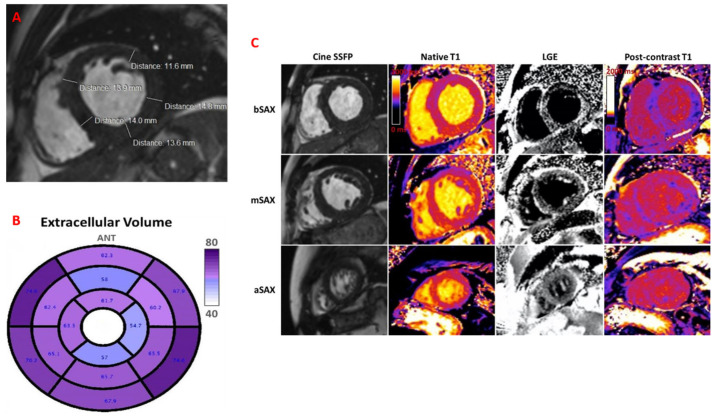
Cardiac magnetic resonance images. (A) Short-axis view showing left ventricular hypertrophy (IVS = 13.9 mm, AWT = 13 mm, LWT = 14.8 mm, PWT = 14 mm). (B) Extracellular volume (ECV) bull’s eye plot evaluated by cardiac magnetic resonance, using a 16-segment model, displaying a progressive decrease of the ECV from base to apex, explaining the substrate of apical sparing deformation pattern. (C) Short axis views at basal (bSAX), midventricular (mSAX) and apical (aSAX) levels showing concentric left ventricle hypertrophy in SSFP cine images (the first column), with diffuse elevation in native T1 mapping (the second column), transmural hyperenhancement, more pronounced at the basal and midventricular levels, with a dark blood pool in LGE images (the third column) and diffuse reduction in post-contrast T1 mapping (forth column). SSFP: Steady-state free precession; LGE: late gadolinium enhancement.
